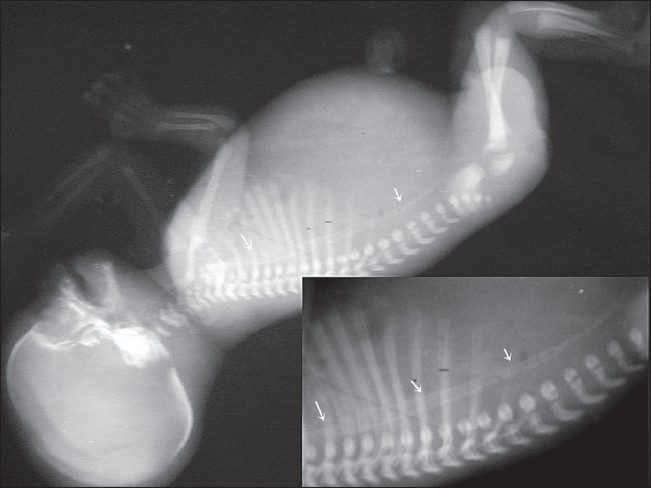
Lateral infantogram radiograph shows calcification of the aorta and iliac vessels (arrows)
